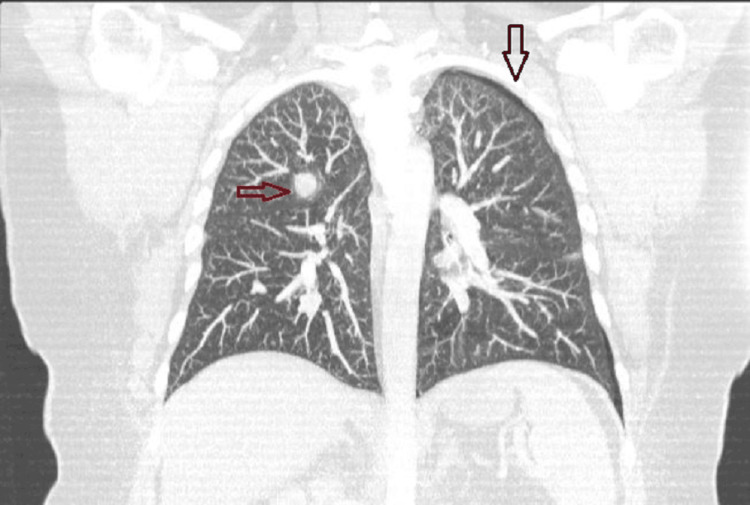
CT chest angiogram demonstrating right nodular mass (red arrow) and left small pneumothorax (black arrow) CT: computed tomography
